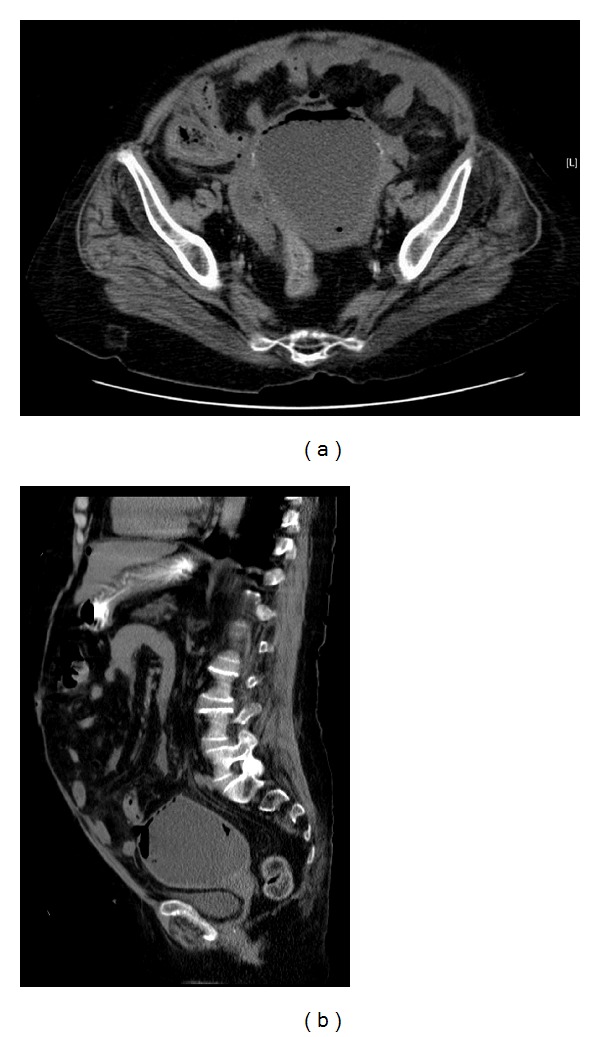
Transverse (a) and sagittal (b) views of noncontrasted-enhanced computed tomography (CT) scan showing a significantly distended fluid-filled uterus measuring 10 × 7.8 × 10 cm, in addition to a single focus of perforation involving the uterine fundus and associated with presence of free air within the nondependant area. No evidence of ascites or pelvi-abdominal lymphadenopathy was identified.
